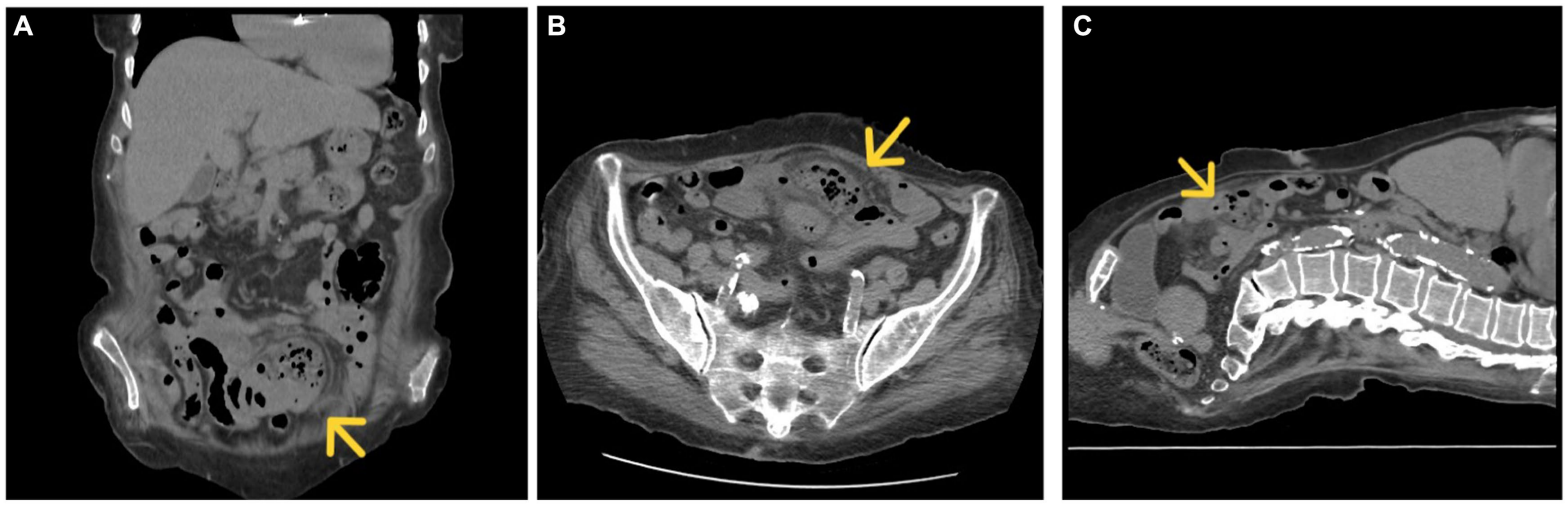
CT, coronal (A), axial (B), and sagittal section (C) inflammatory block marked with a yellow arrow.
